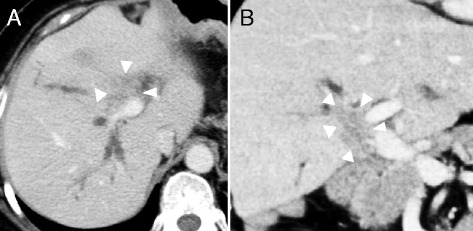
Computed tomography of the relapse of cholangiocarcinoma. a, b Intrahepatic bile duct is dilated, and low-density tumor is appreciated at the hilum of the liver. *Arrows* represent the low density tumor which is the relapse of residual cancer cells at surgical margin of biliary tract
